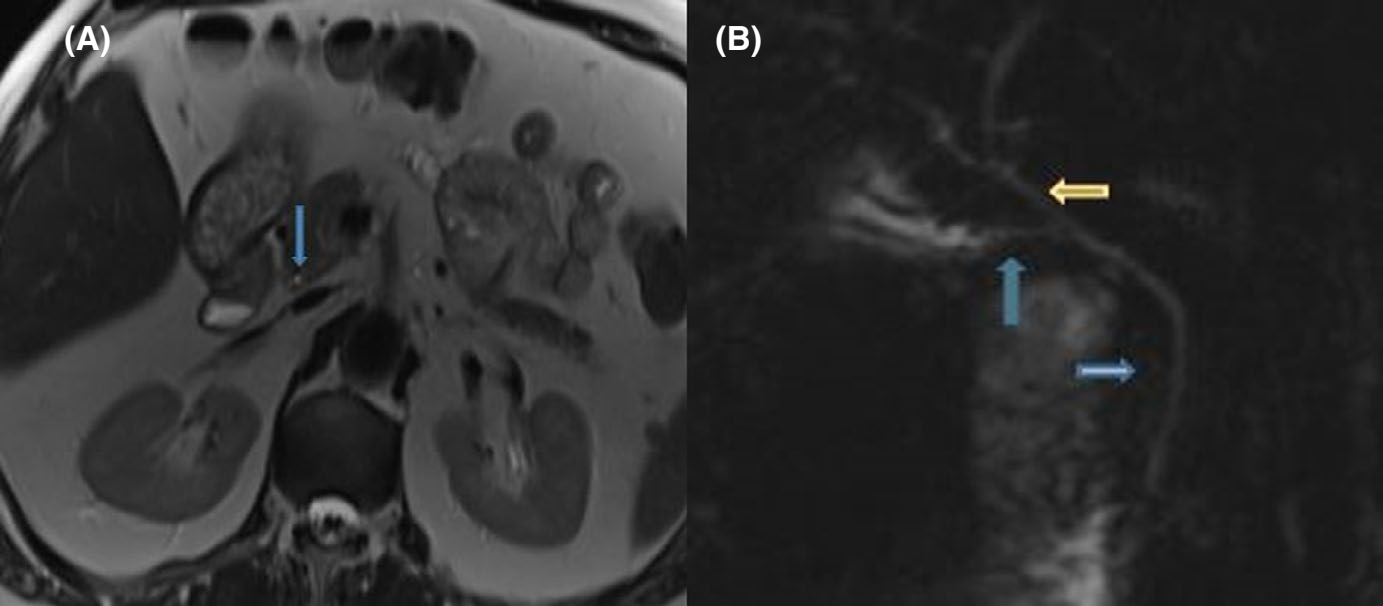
(A) axial magnetic resonance imaging (B) magnetic resonance cholangiopancreatography imaging. Extrahepatic and intrahepatic bile ducts appear normal in the patient. (Blue thin arrow: choledoch, yellow arrow: common bile duct, thick blue arrow: cystic duct)
